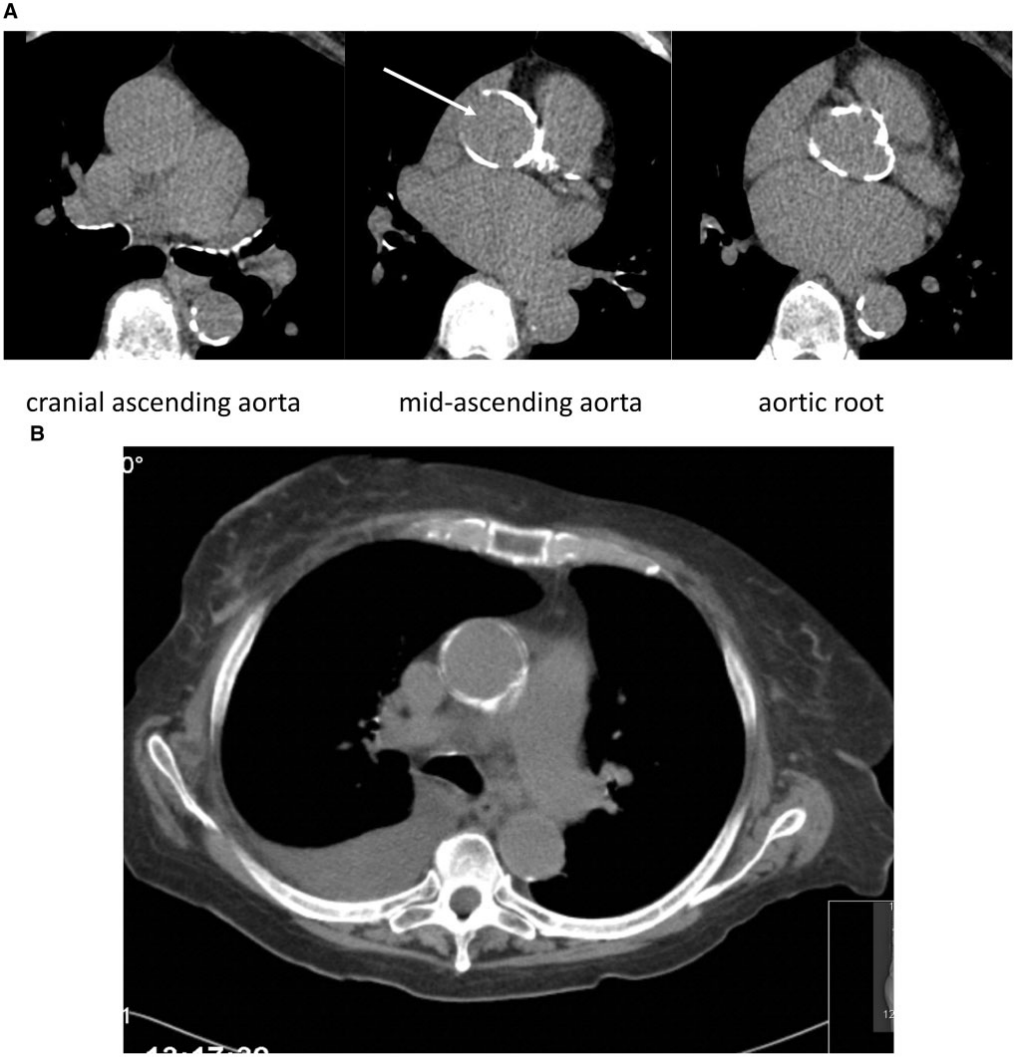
(A) Computed tomography showing the calcifications at different level of the ascending aorta and the aortic root with the ‘calcium-free window’ on the right lateral wall (white arrow), where the aorta was opened for aortic valve replacement. (B) Coronal view of the ascending aorta.
